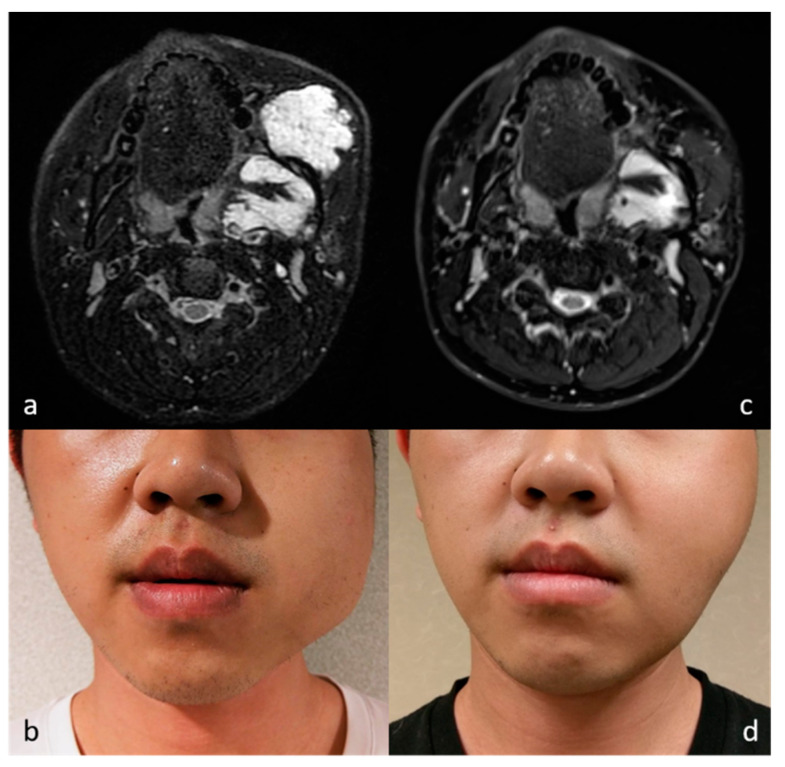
MR images of patient 2 before (a) and after (c) RFA show almost total volume reduction of the targeted venous malformation in the left masticator space. The lesion in the deep layer remained stable in size. Photographs before (b) and after (d) RFA show satisfactory improvement of facial asymmetry (cosmetic scale 4 → 3). Residual facial asymmetry was due to bone remodeling and deep-seated lesions.
